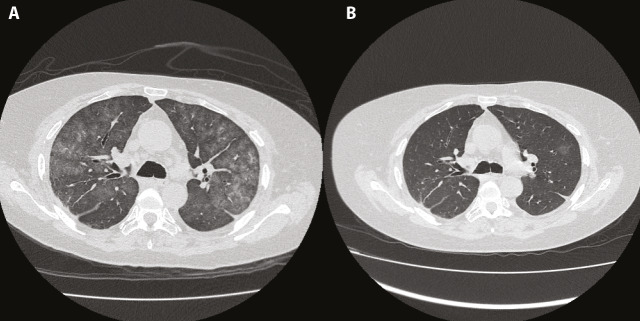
High-resolution computed tomography scan of the chest. (A) Pre-treatment scan showing
